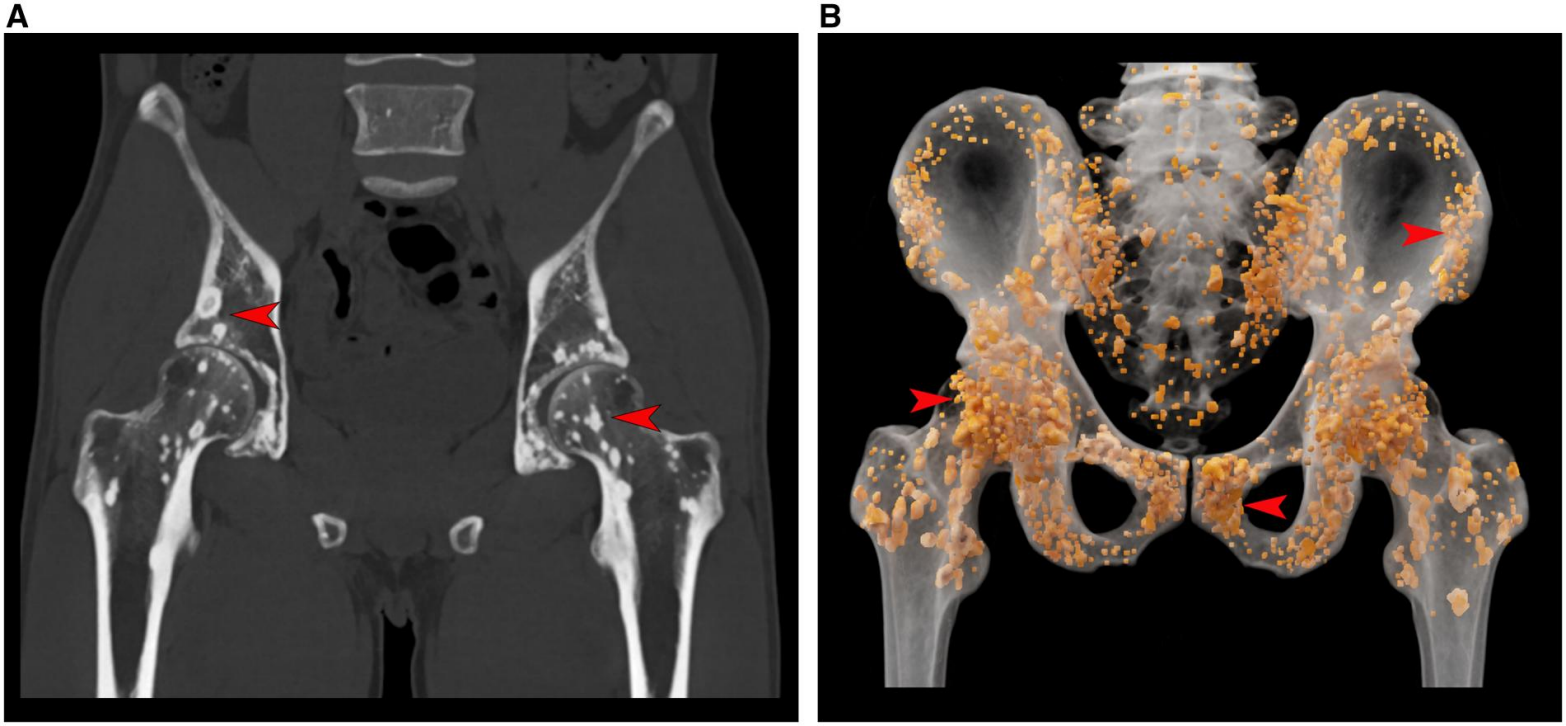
Images from a 26-year-old man with osteopoikilosis. (A) Pelvic CT scan in coronal view revealed extensive punctate high-density shadows within the pelvic region and bilateral proximal femurs (arrowheads). (B) Coronal cinematic rendering images, rendered individually for each bone lesion, demonstrated osteopoikilosis in 3D (arrowheads)
